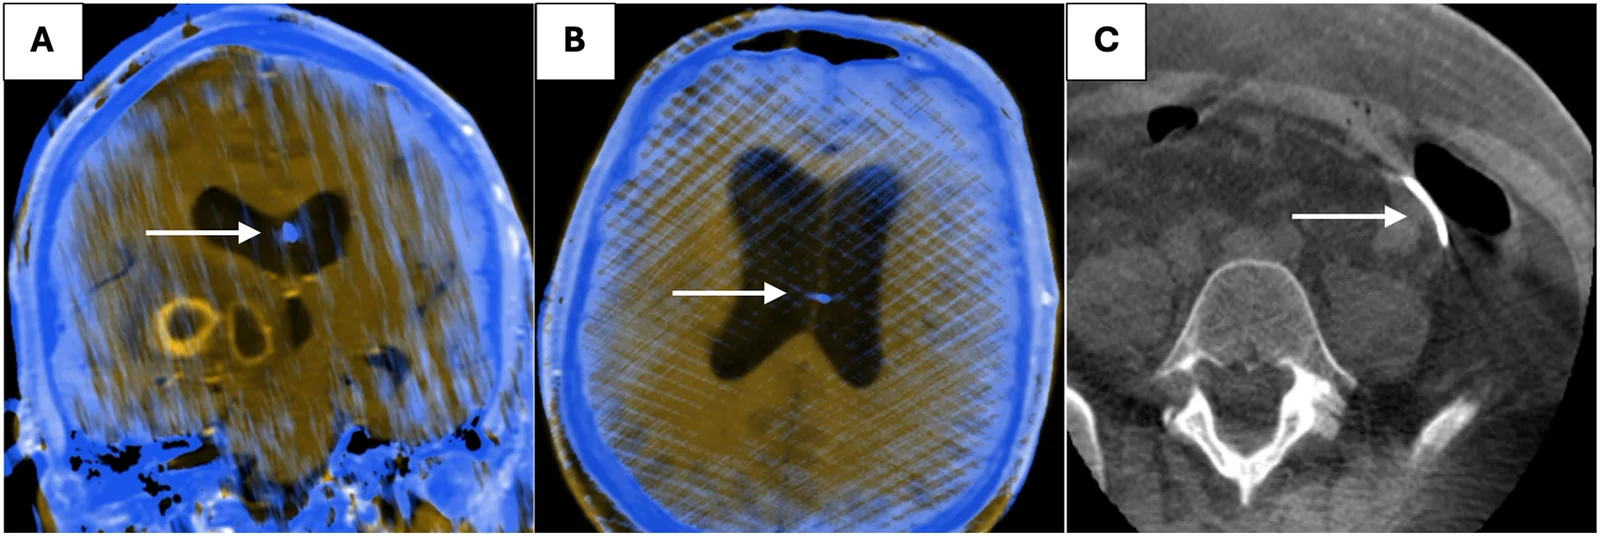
Intraoperative CBCT of the brain fused with preoperative MRI (A, coronar; B, axial) demonstrating cross-over positioning of the ventricular catheter, which prompted immediate intraoperative revision. A second scan confirmed correct intraventricular position of the catheter. Intraoperative CBCT of the abdomen (C) demonstrating intraperitoneal course and correct positioning of the distal catheter (white arrow).
